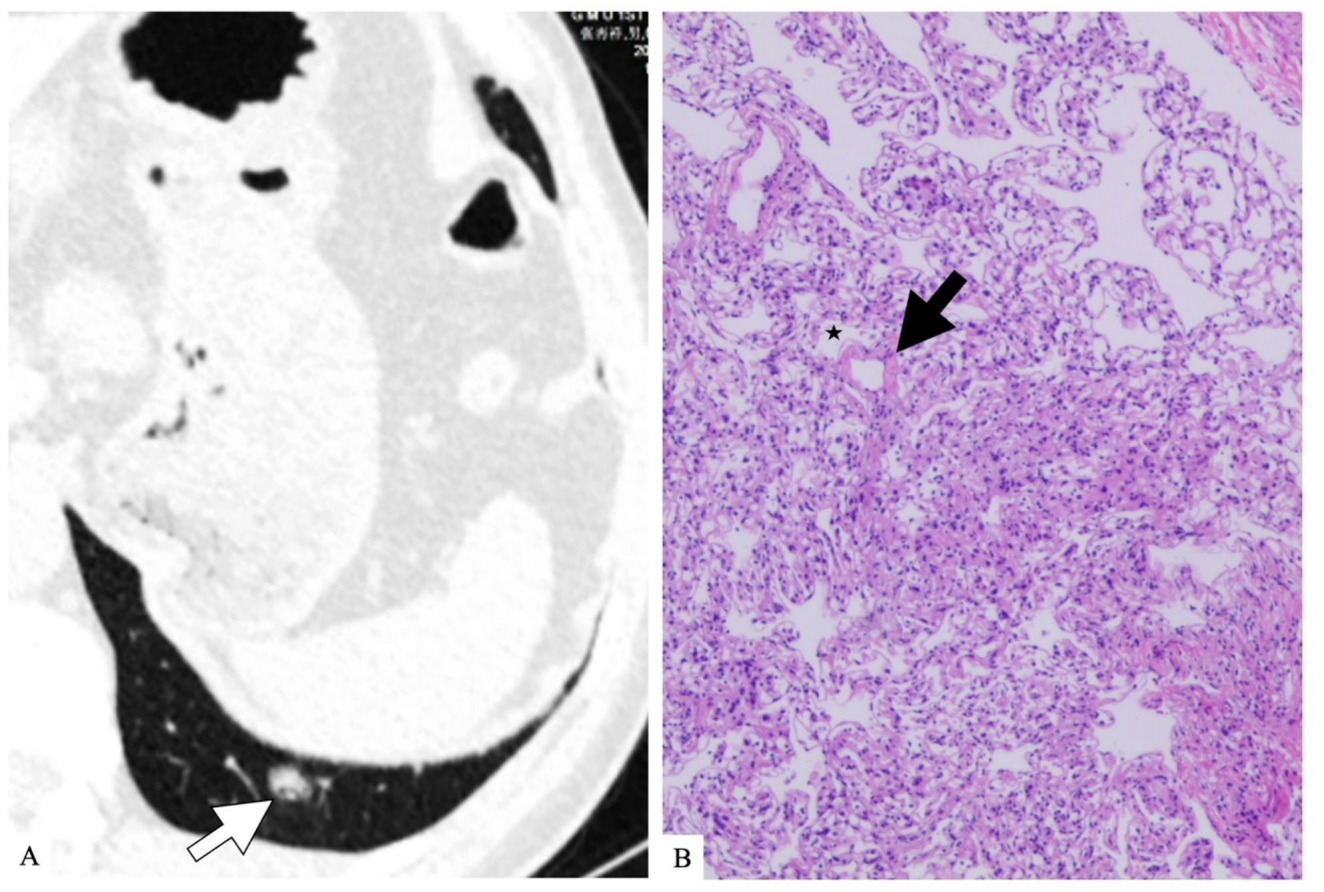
Example of radiological and pathological images of perivascular lucency in a solitary pulmonary capillary hemangioma (SPCH). A): transverse CT image of a 64-year-old woman who had a solid SPCH in the left lower lobe with perivascular lucency (arrow). B): pathological image of the same patient revealed a dilated pulmonary vein (arrow) with adjacent relatively spared capillary proliferation (star) in a solid SPCH
